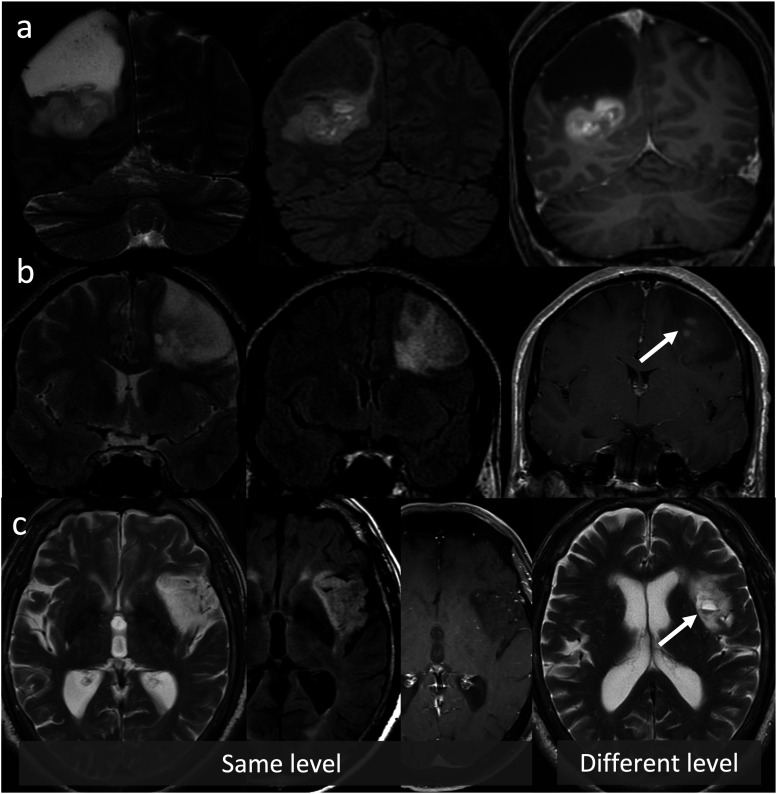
Lesions that show T2FMM in their subregions. (a) IDH-mutant, 1p/19q non-codeleted glioma in a 43-year-old female. While the superior subregion of the mass demonstrates T2FMM, the inferior subregion has heterogenous signal on T2WI (left column), lacks suppression on FLAIR (middle column), and diffusely enhances (right column). (b) IDH-mutant, 1p/19q codeleted glioma with partial T2FMM in a 46-year-old male. While the superficial and peripheral subregions demonstrate T2FMM, the deeper and more central portion demonstrates heterogenous signal on T2WI, lacks suppression on FLAIR, and shows patchy enhancement (arrow). (c) IDH-mutant, 1p/19q codeleted glioma with partial T2FMM in an 81-year-old male. While the more inferior subregion demonstrates T2FMM, the more superior subregion shows small cysts, one of which has dependent-layering susceptibility compatible with blood products (arrow).
